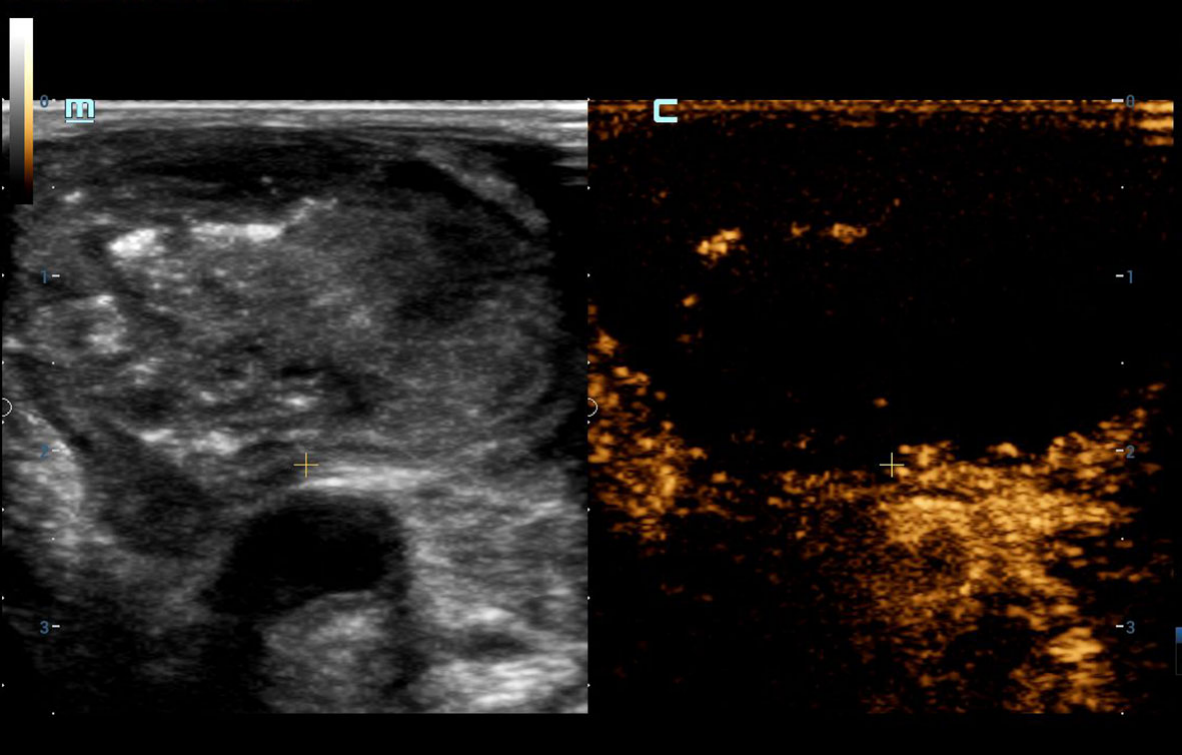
No contrast enhancement in left cervical mass according to postoperative ultrasonography.
